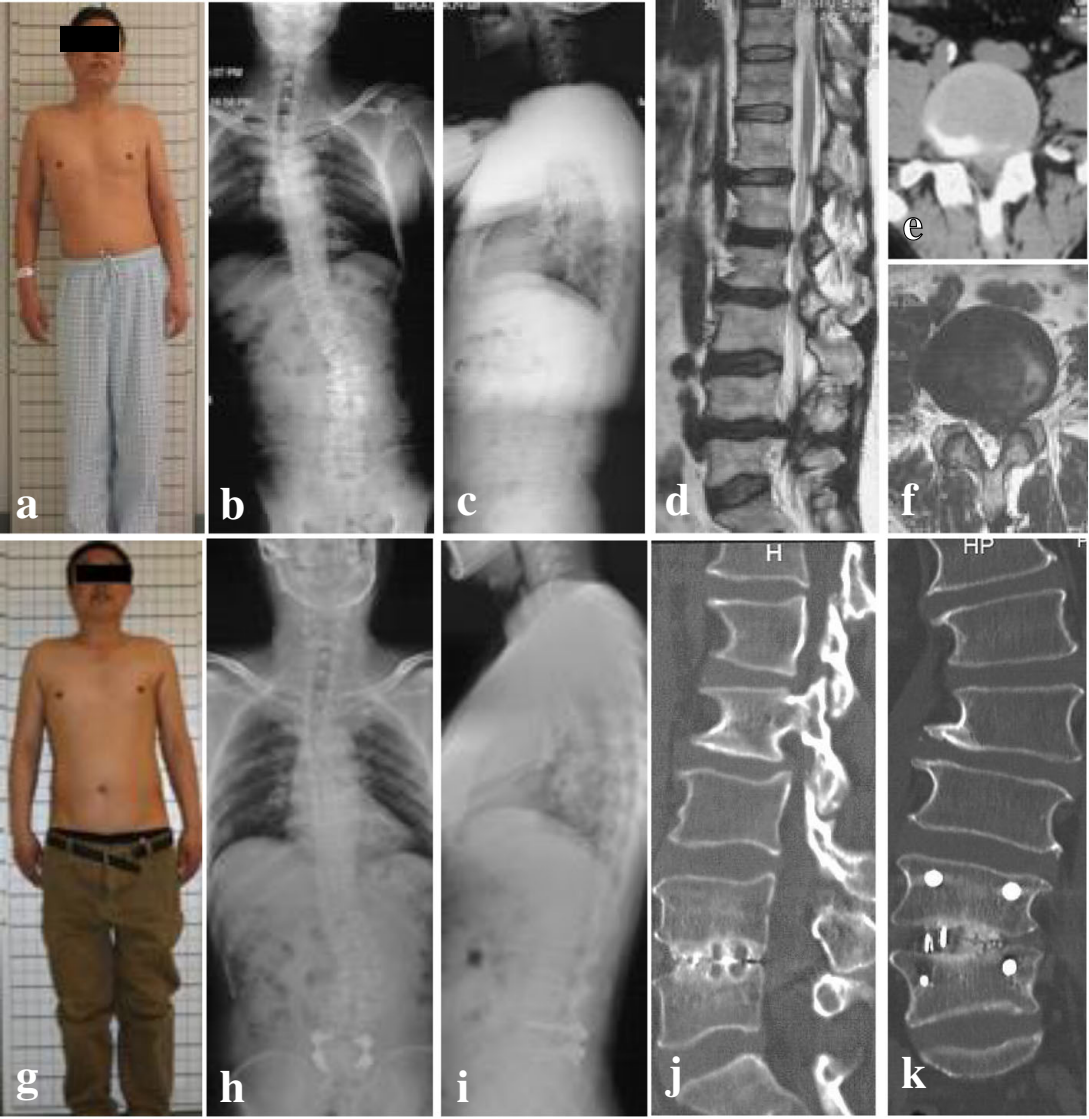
A 65-year-old male patient suffering from adult lumbar degenerative scoliosis. The main complains were severe back and left leg pain complicated with intermittent claudication. a Preoperative view photograph showed that the trunk tilt to the left is obvious. b, c Preoperative X-ray showed the Cobb angle was 32°, and the coronal was imbalanced. d, e, f Preoperative CT and MRI showed the L4/5 disc herniation. g The view photograph of the 2-year follow-up showed that the trunk tilt is not obvious. h, i The X-ray of the 2-year follow-up showed the Cobb angle was 23°, and the coronal was balanced. j, k The CT of the 2-year follow-up showed grade 1 fusion
